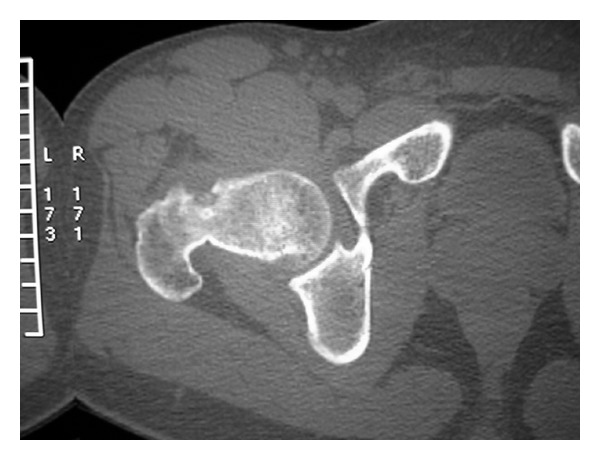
CT scan showing the subperiosteal nidus.
